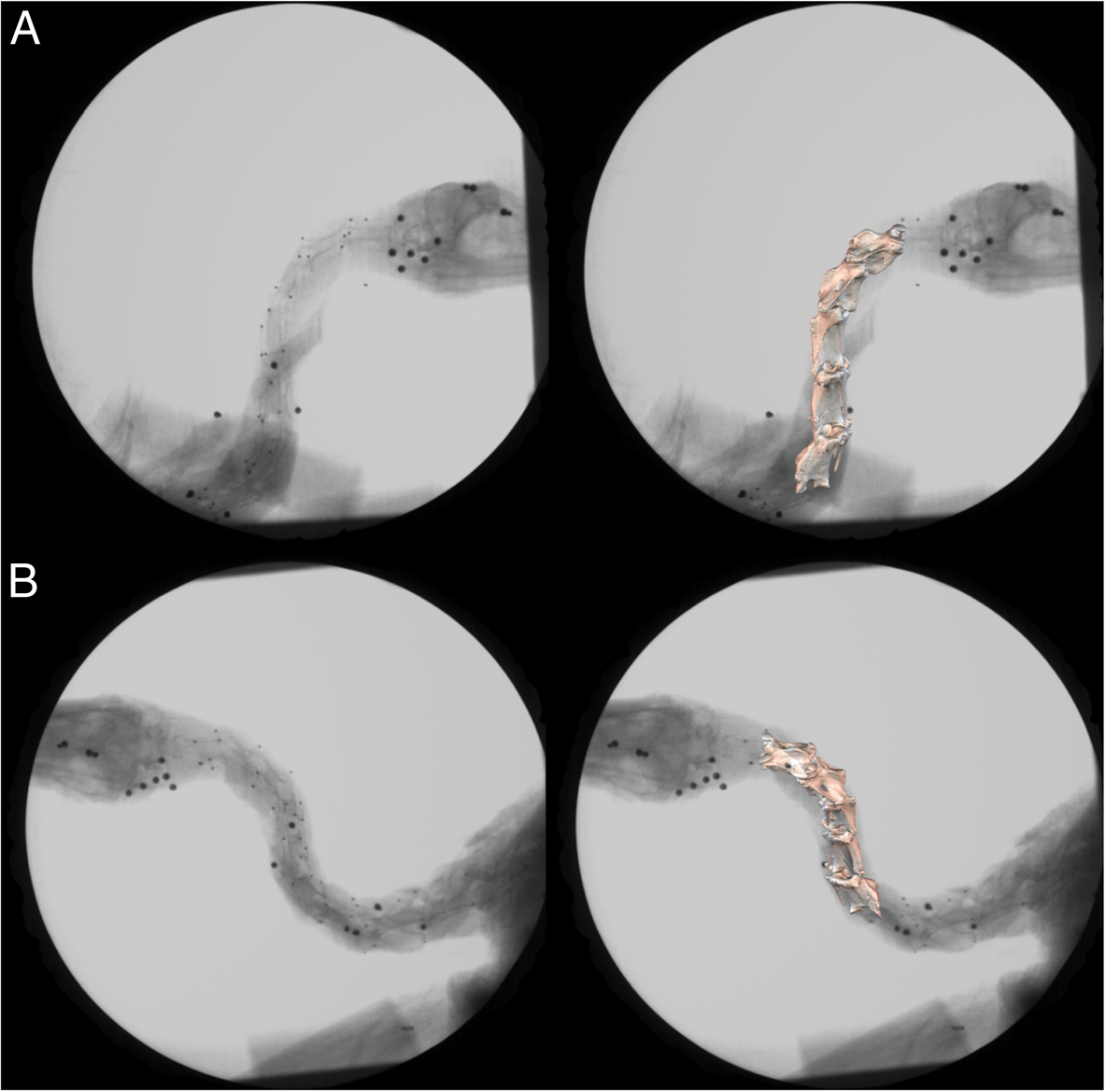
Stills from biplanar X-ray videos. a, b. *Left*: Original frame. *Right*: Frame with reconstructed bone motion overlaid
